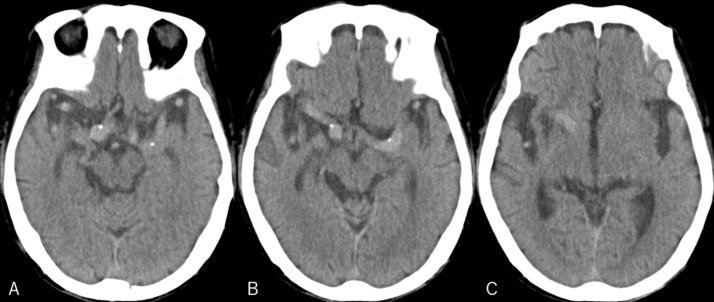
(*A-C*) Non-contrast axial computed tomography taken 3 months later showing resolution of the intracerebral hemorrhage.
